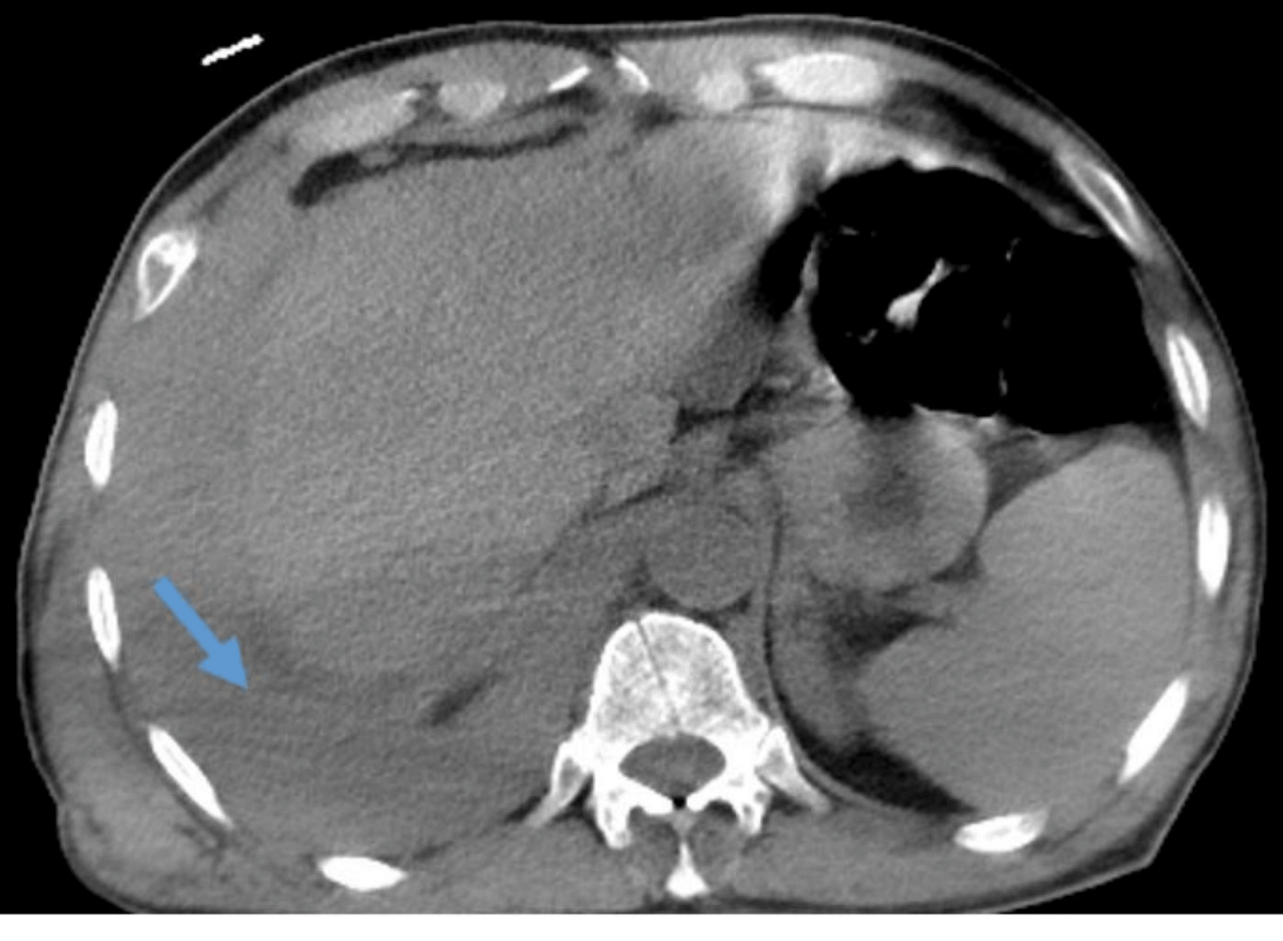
A non-contrast-enhanced abdominal CT scan reveals perihepatic effusion and fluid accumulation in the supramesocolic compartment.
